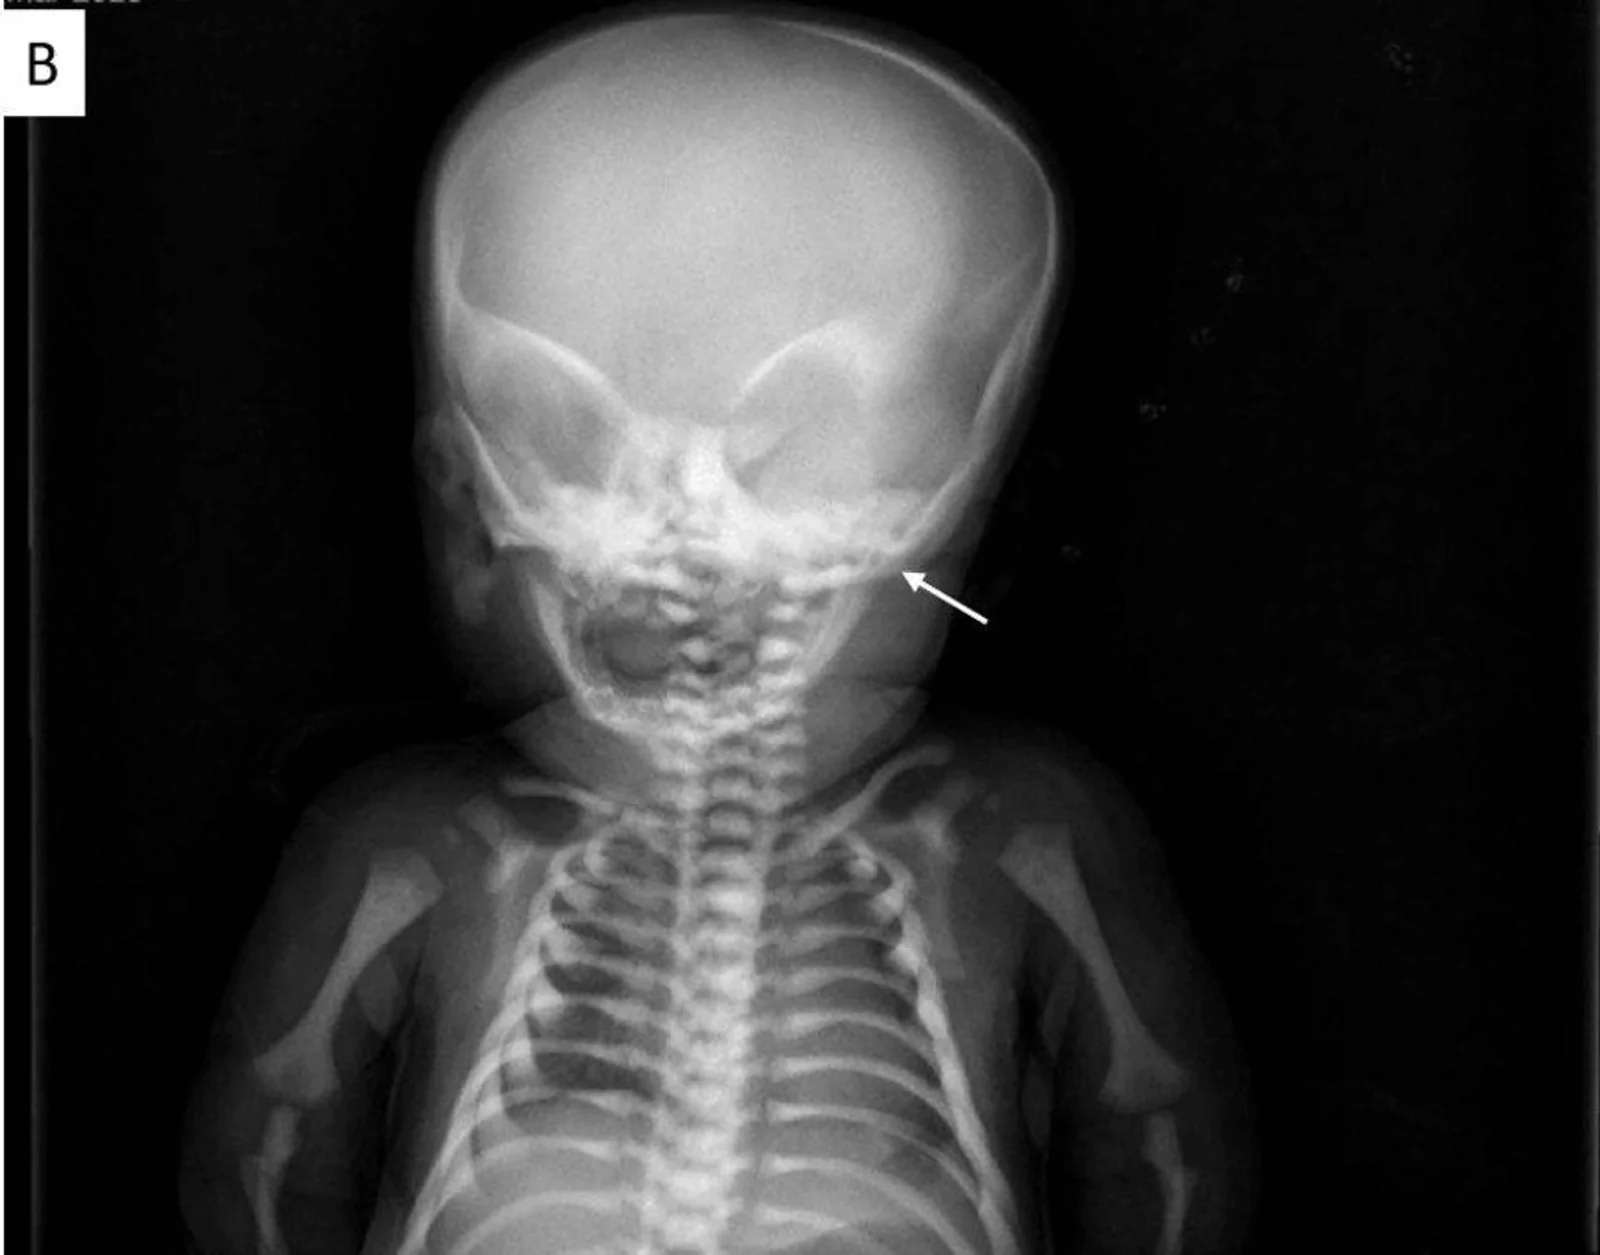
Anteroposterior skull radiograph demonstrating diffuse calvarial sclerosis with increased skull base density (white arrow), compatible with osteopetrosis.
